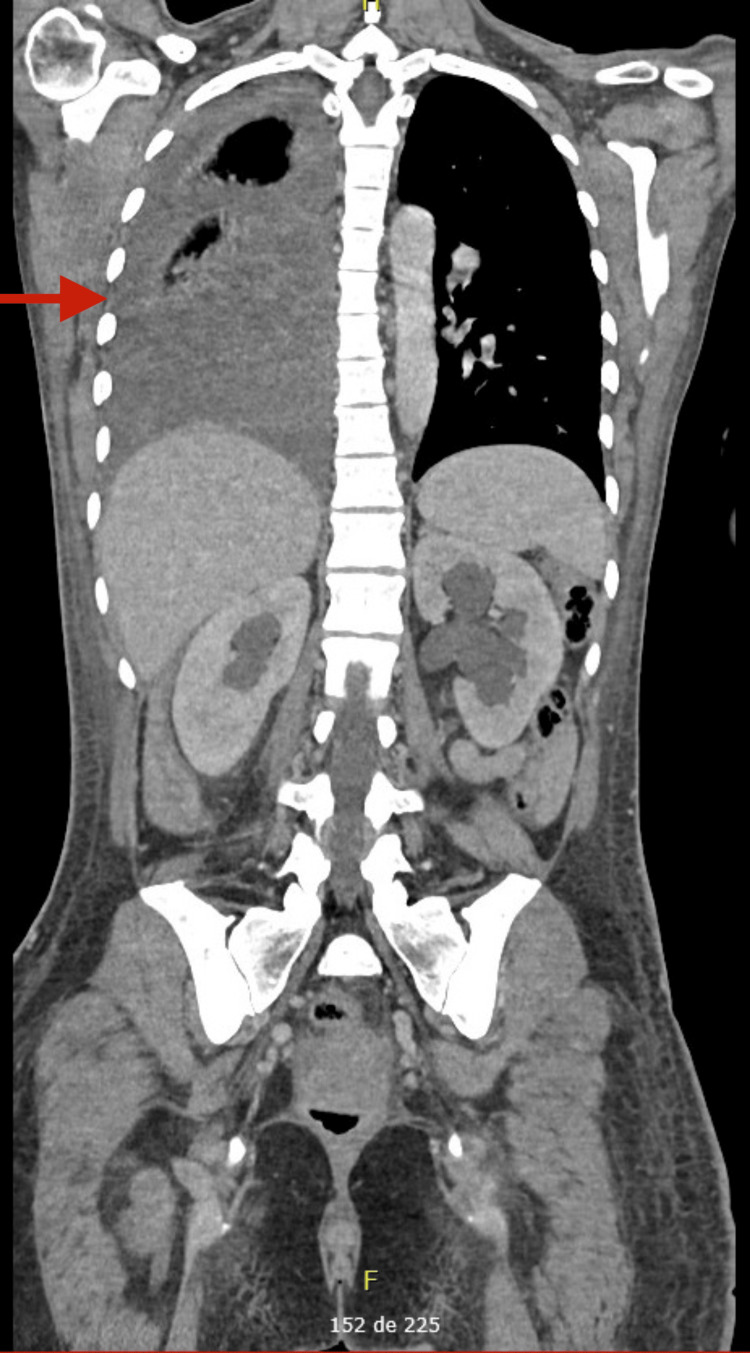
Thoracic CT scan (coronal plane) demonstrating the hemothorax (red arrow)
